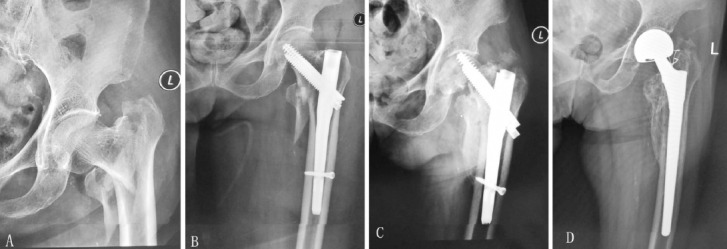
Anteroposterior radiograph of a 73-year-old female showing intertrochanteric fracture treated with intramedullary fixation (A and B). Nonunion result in cut-out of the internal device at one year after operation (C). Anteroposterior radiograph two year after salvage uncemented bipolar long-stem hemiarthroplasty showing good prosthesis position and osteointegration (D).
